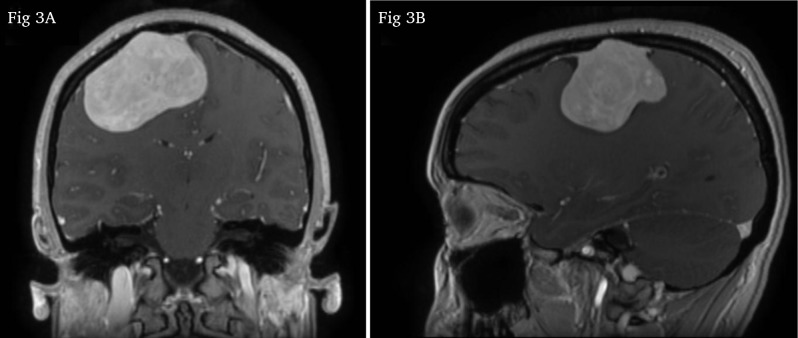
Coronal and sagittal T1-weighted images after intravenous administration of gadolinium contrast clearly demonstrate the dural tail sign.
